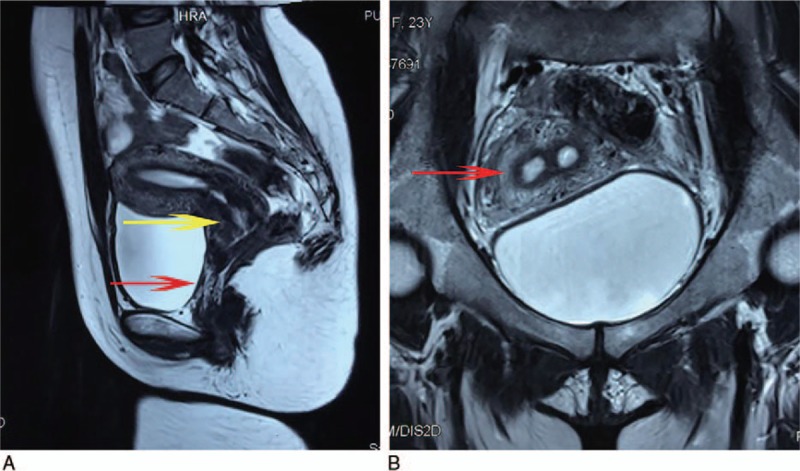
Magnetic resonance imaging scan showing (A) distinct cervices (yellow arrow) and no obvious low vagina (red arrow). (B) The septum is causing exaggerated separation of the cavities (red arrow).
